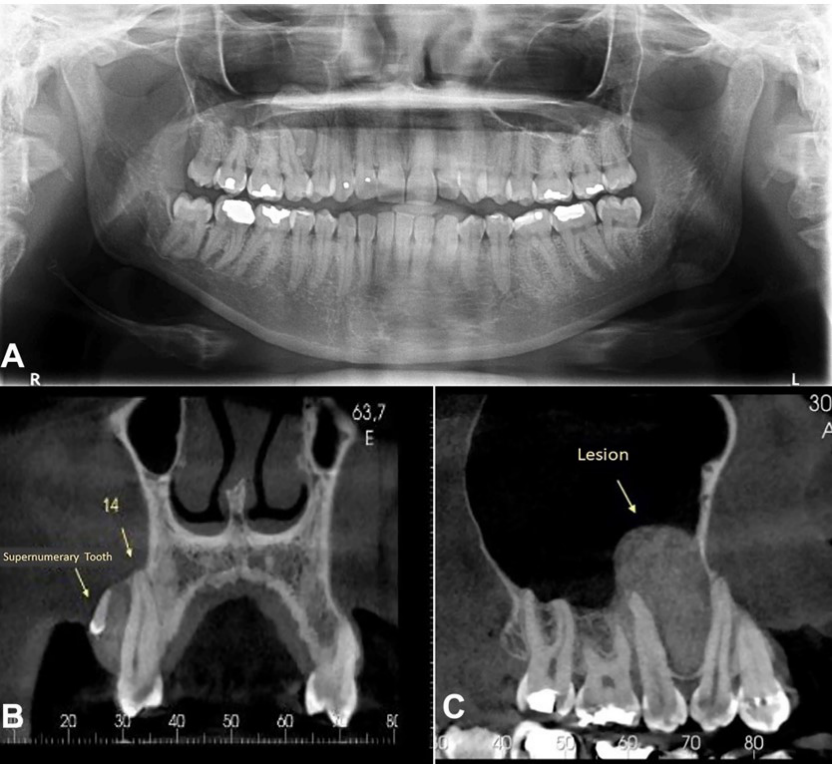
Imaging features of the lesion. A – Panoramic radiograph showing a unilocular lesion between the roots of right upper premolars and the presence of denticle-like mineralized material; B – Coronal view of cone-beam computed tomography (CBCT) image. Isodense lesion associated with upper right first premolar. Note the presence of a micro-tooth inside the tumor; C – Sagittal view of CBCT image. Isodense oval lesion in the right maxilla extending into the maxillary sinus and causing displacement of the roots of right upper premolars.
